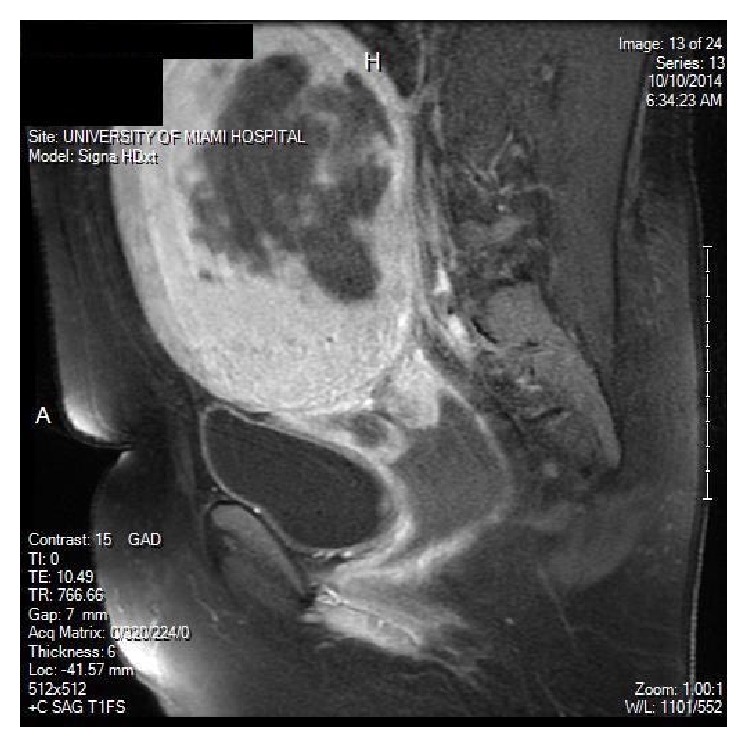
MRI showing a large central necrotic fibroid.
